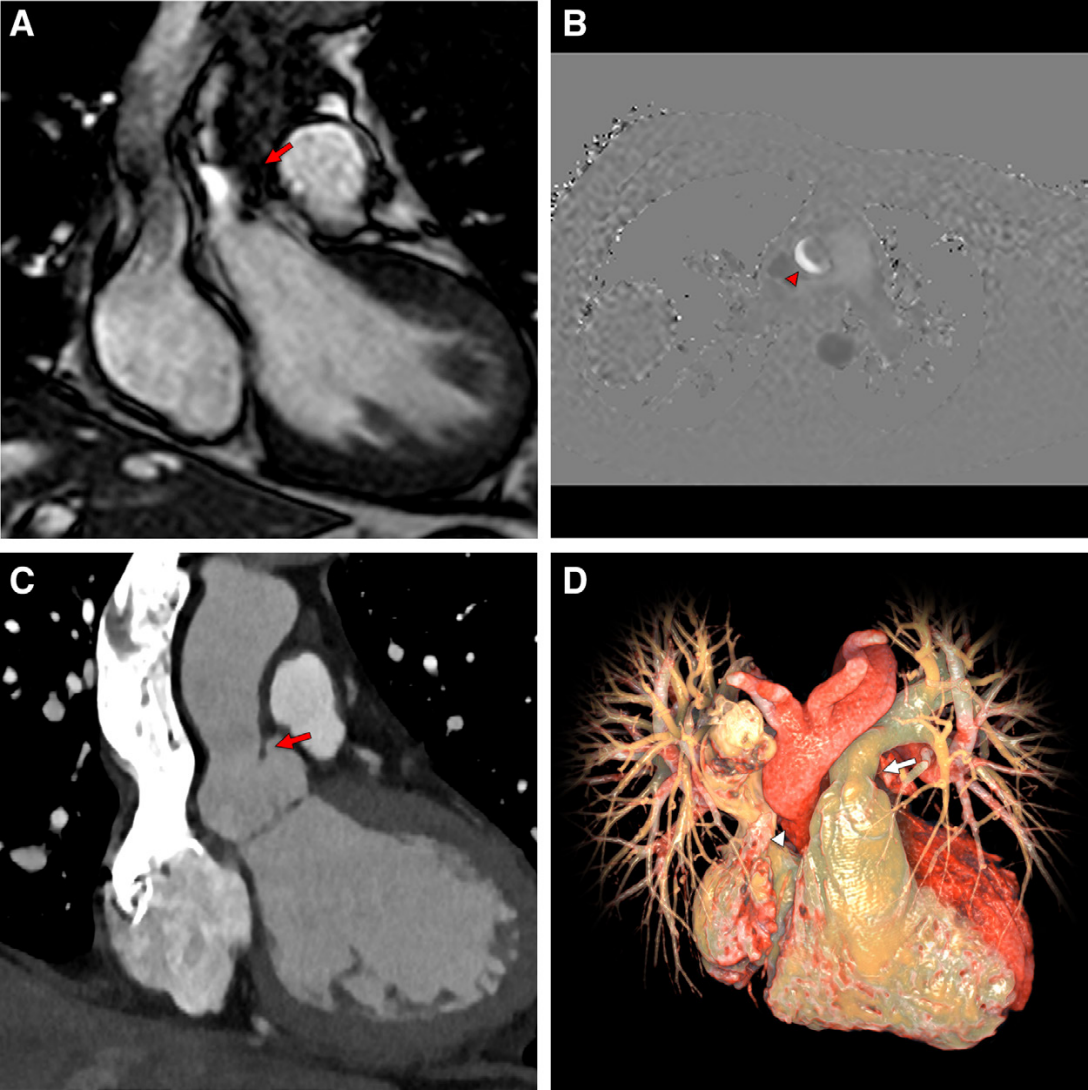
Multimodality imaging assessment of supravalvular aortic stenosis in a 37-year-old man. A, Cardiac magnetic resonance imaging (MRI) balanced steady-state free-precession sequence along the left ventricular outflow tract, showing turbulent blood flow above the aortic valve during systole (red arrow). B, Cardiac MRI through-plane phase-contrast velocity mapping obtained at the sinotubular junction level shows a crescent-like appearance of the aortic flow (red arrowhead). C, Cardiac computed tomography angiography (CTA) multiplanar reconstruction along the left ventricular outflow tract confirms the presence of a supravalvular aortic membrane (red arrow), with hourglass stenosis of the proximal ascending aorta. D, Cardiac CTA photorealistic volume rendering image showing the hourglass appearance of the proximal ascending aorta (white arrowhead) and the mild supravalvular pulmonary stenosis (white arrow).
